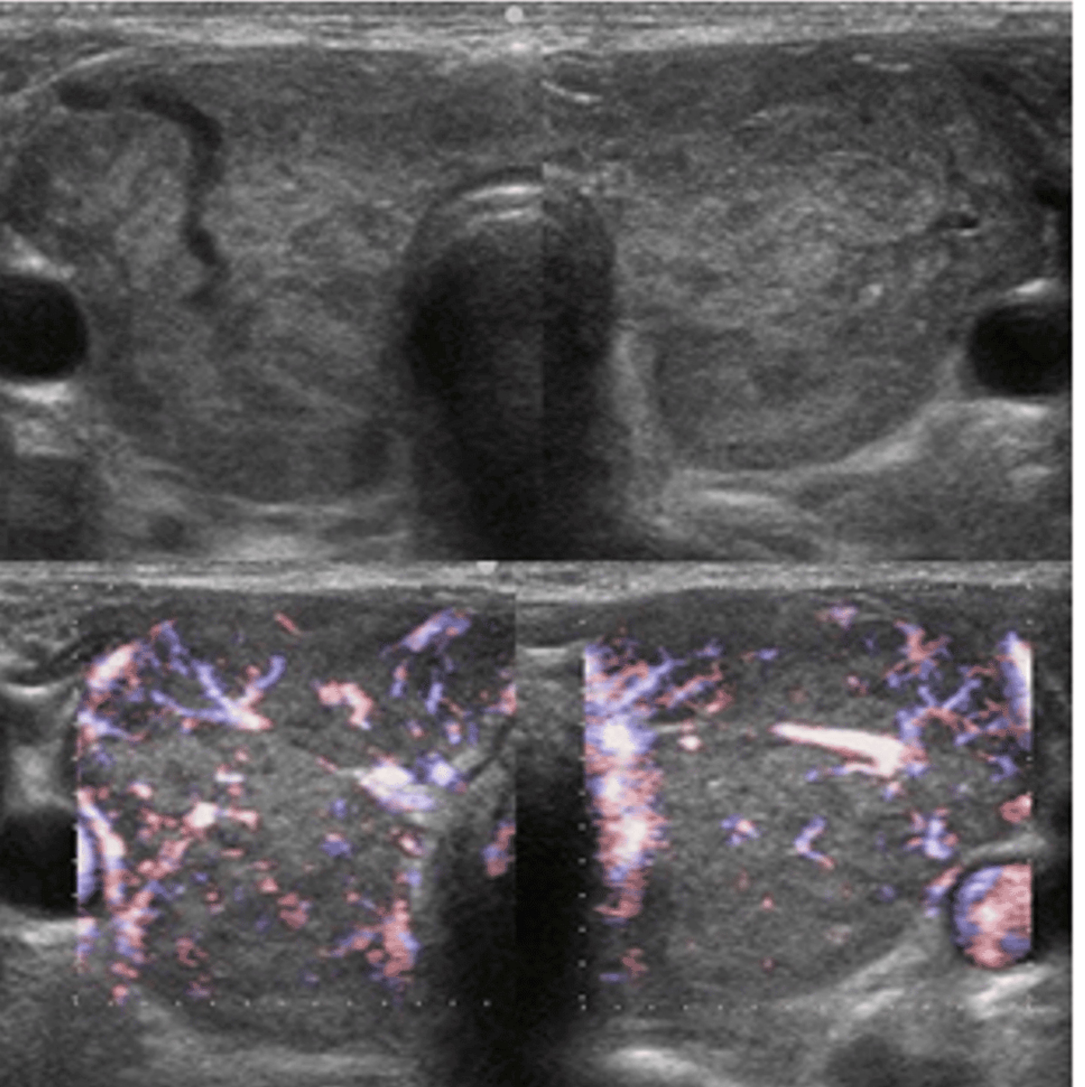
Ultrasonography on admission.
